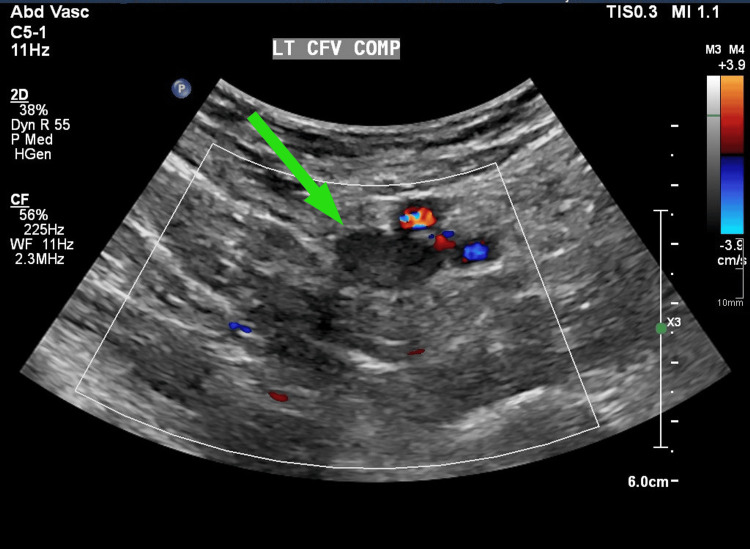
Left common femoral vein compression (CFV COMP) with thrombus within on ultrasound compression venography
